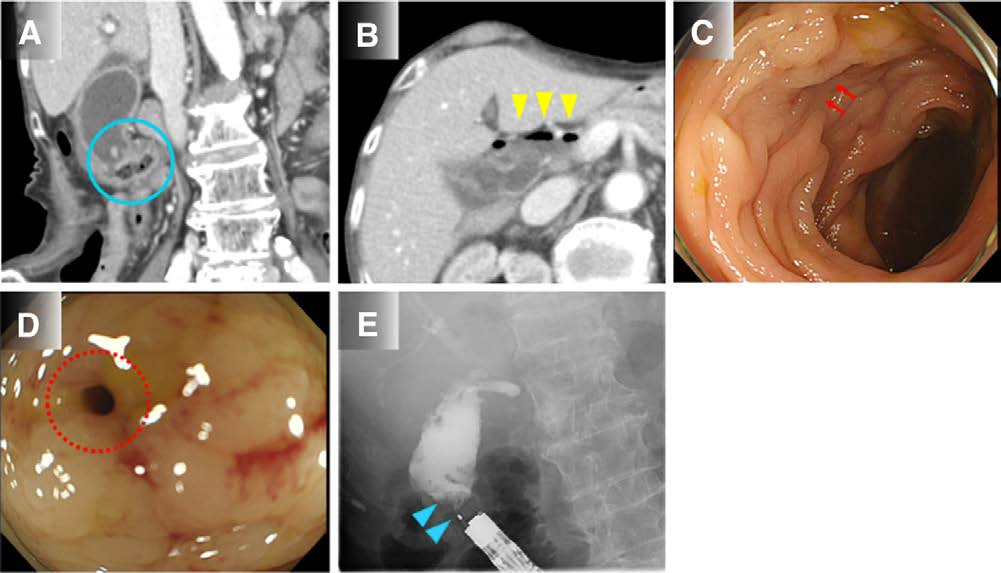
Patient imaging before endoscopic closure. (A) Abdominal contrast-enhanced computed tomography (CT). A suspected fistula is seen between the gallbladder and the transverse colon (blue circle). (B) Abdominal CT showed pneumobilia from the gallbladder to the common bile duct (yellow arrowhead). (C) Colonoscopy shows edematous changes in the mucosa of the transverse colon near the hepatic flexure, and a fistula could be seen between the folds (red arrow). (D) The fistula with a diameter of 3–4 mm was confirmed (red dotted circle). (E) When contrast medium was injected into the fistula, the gallbladder and bile duct were easily visualized (blue arrowhead).
